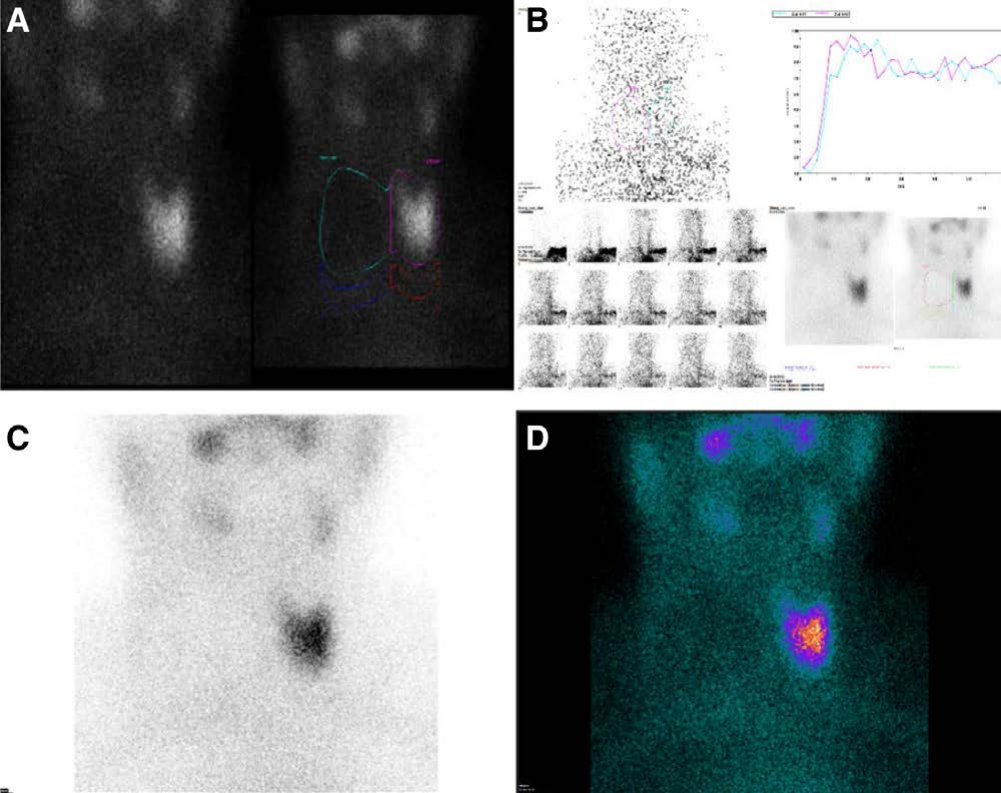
(A–D) Thyroid scintigraphy in February 2022: Parameters include thyroid technetium uptake at 0.5% and thyroid-to-background ratio (T/B) at 1.7. The lack of technetium uptake in the right lobe and isthmus denotes a “cold nodule,” accompanied by diminished global ^99m^TcO4^−^ uptake in the thyroid.
